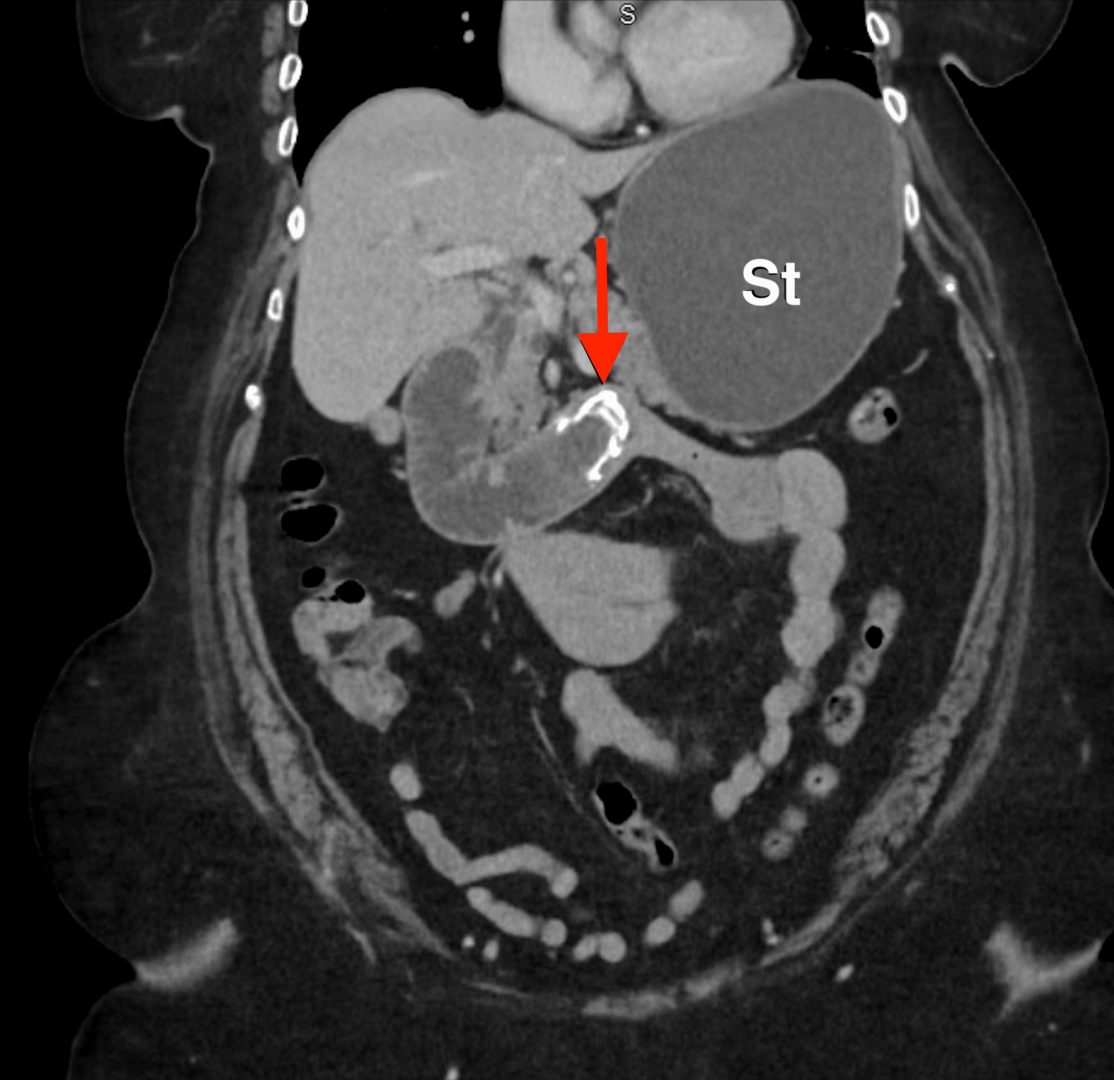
Coronal contrast-enhanced CT of the abdomen demonstrating a hyperdense calculus within the duodenal lumen (arrow), consistent with an ectopic gallstone causing proximal obstruction with stomach (St) distention.
